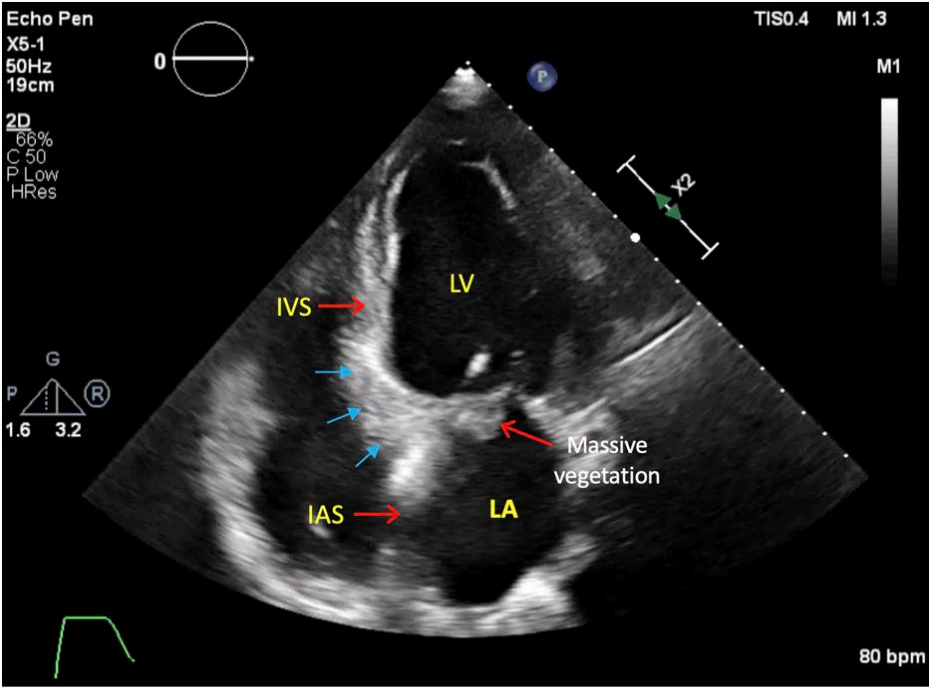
Transthoracic echocardiography, four-chamber view. On admission during the second hospitalization in 2021. Blue arrows point on thickening of the interatrial and interventricular septum. IAV, interatrial septum; IVS, interventricular septum; LA, left atrium; LV, left ventricle.
